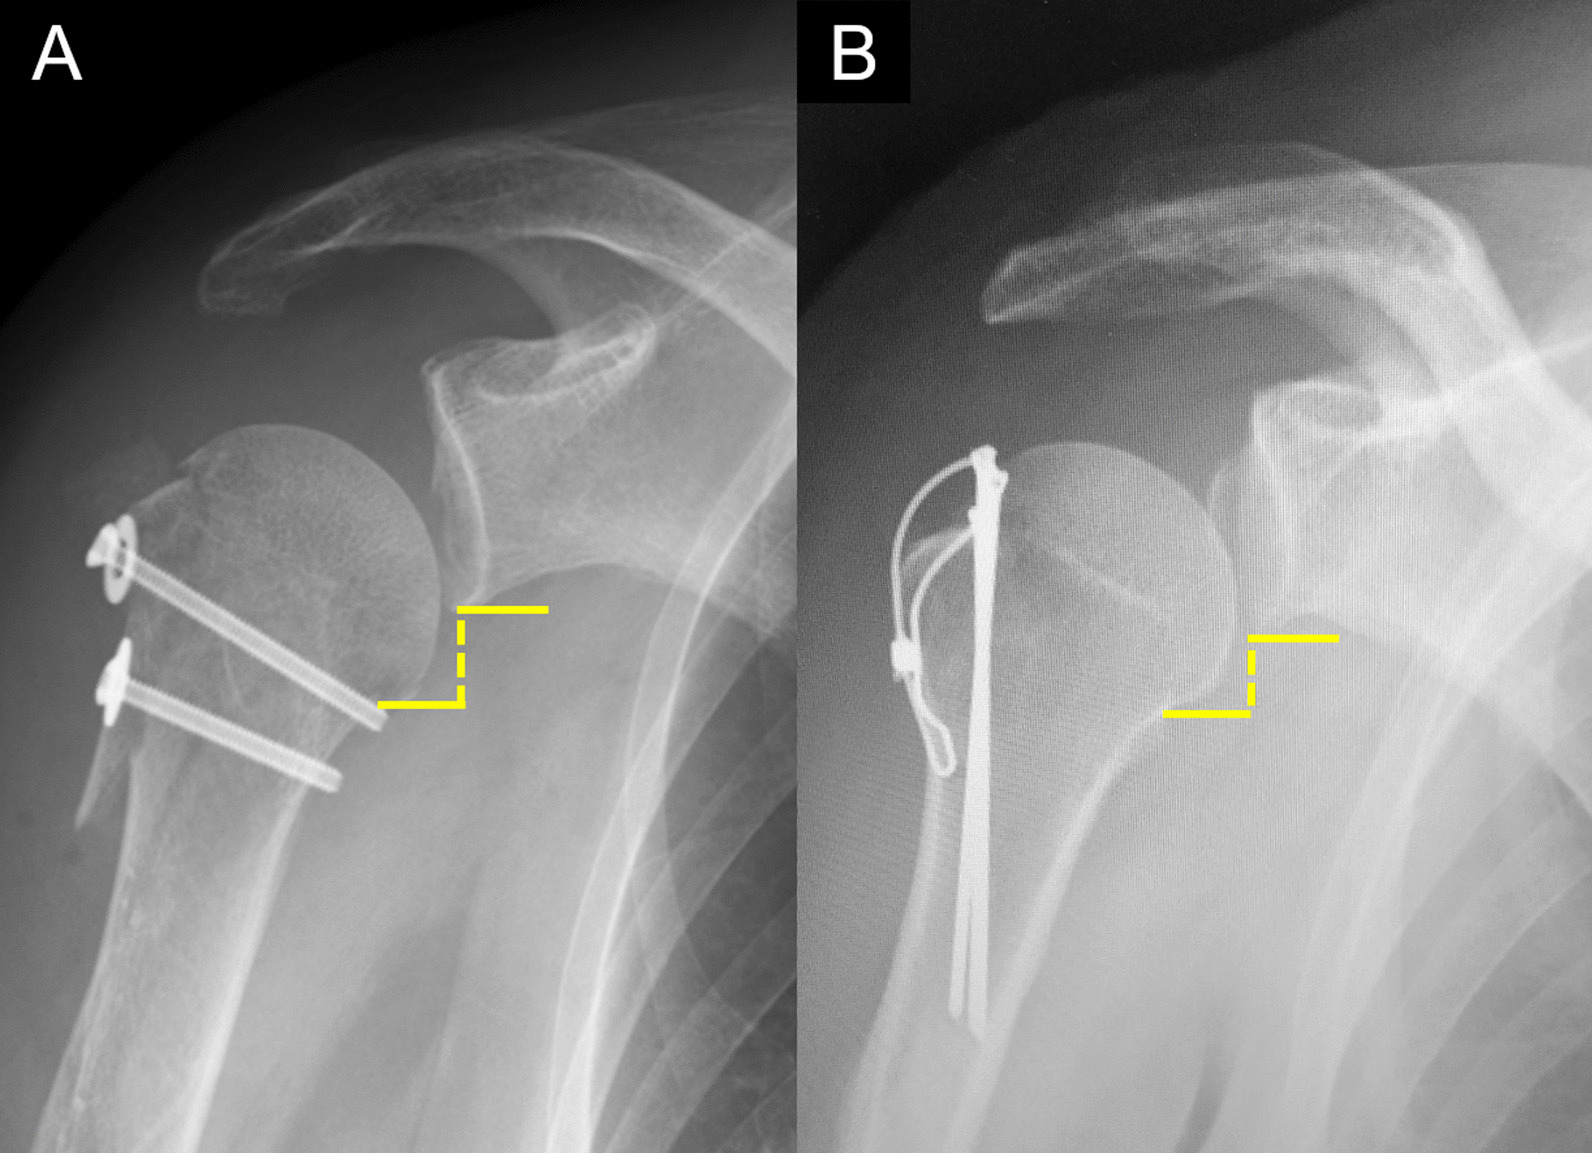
Radiographic assessment of inferior subluxation of the humeral head. A distance of ≥ 1 cm between the humeral anatomical neck level and the glenoid inferior edge level was defined as the presence of humeral head inferior subluxation. Postoperative radiograph images after surgery using a cannulated cancellous screw (A) or tension band wiring (B)
